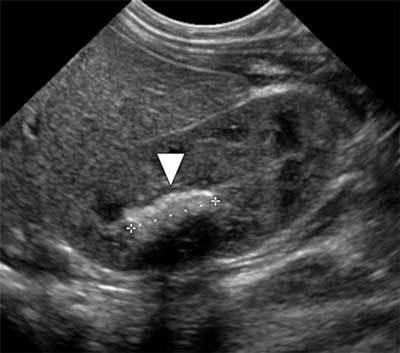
Follow-up ultrasonography shows calcification in the right kidney with posterior shadowing (arrowhead).
